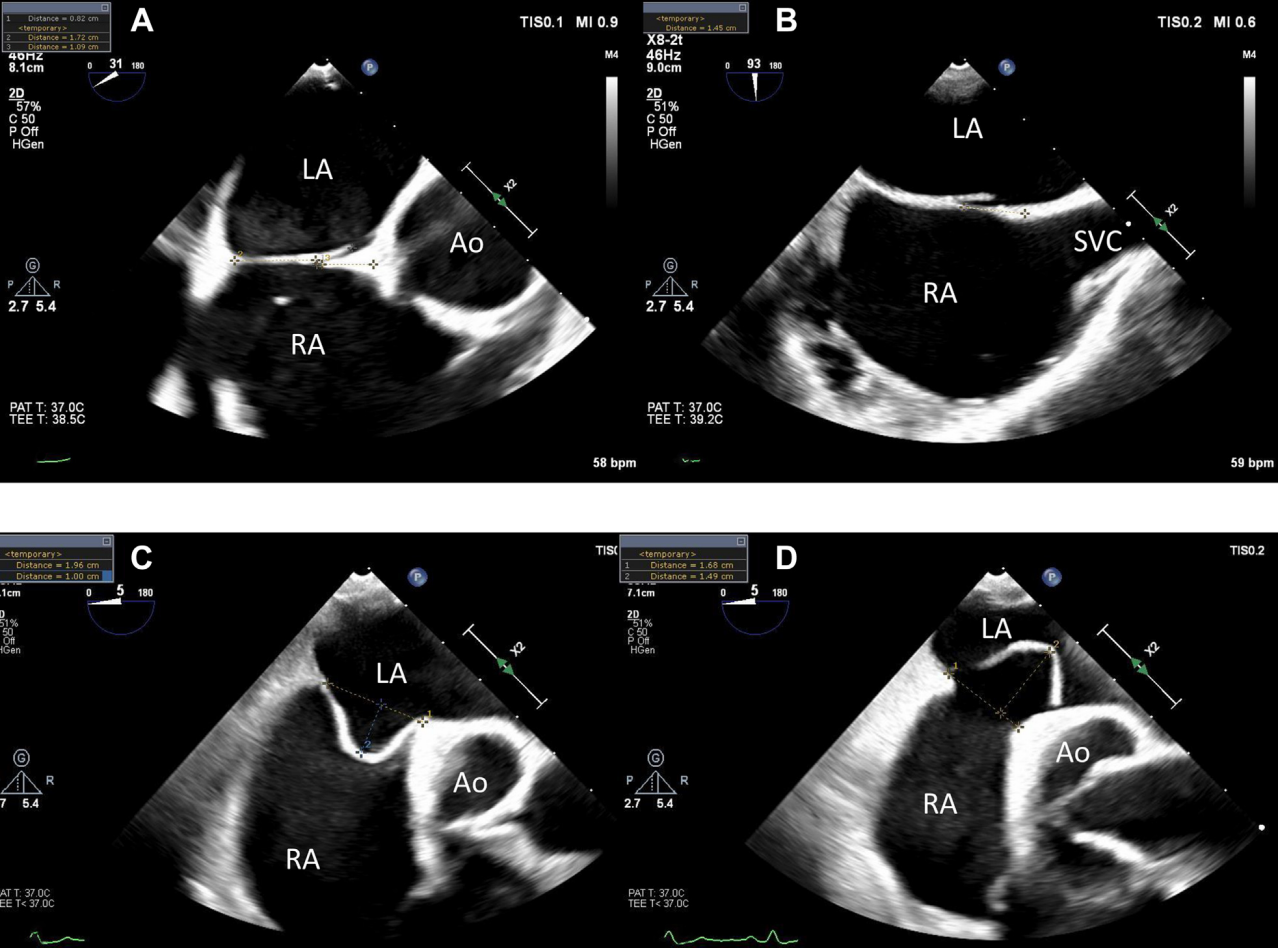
Measurement of Aortic and Superior Vena Cava Distance, and Atrial Septal Excursion by Transesophageal Echocardiogram (A) Mid-esophageal 30° view, with measurement of the posterior and aortic rim. (B) Mid-esophageal 90° view, with measurement of the superior vena cava rim. (CandD) Mid-esophageal 0° to 5° view of an aneurysmal atrial septum, with measurement of septal excursion into the right (C) and left (D) atria. Ao = aorta; LA = left atrium; RA = right atrium; SVC = superior vena cava.
